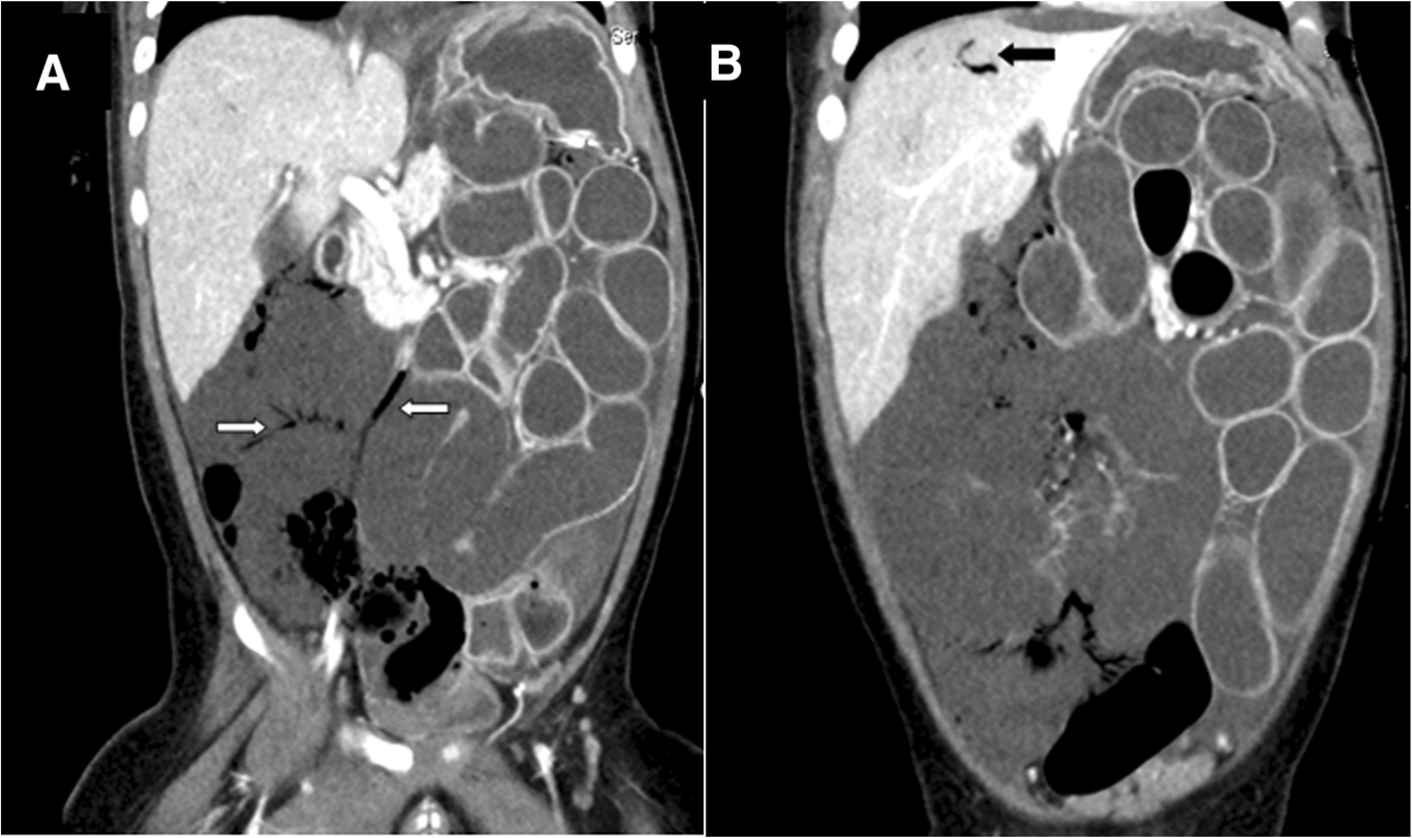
Abdominal CT showed (a) intraluminal pneumatosis intestinalis (white arrows) and poor perfusion of the right abdominal bowel wall; (b) radiolucent lines in the portal venous system (black arrow)
